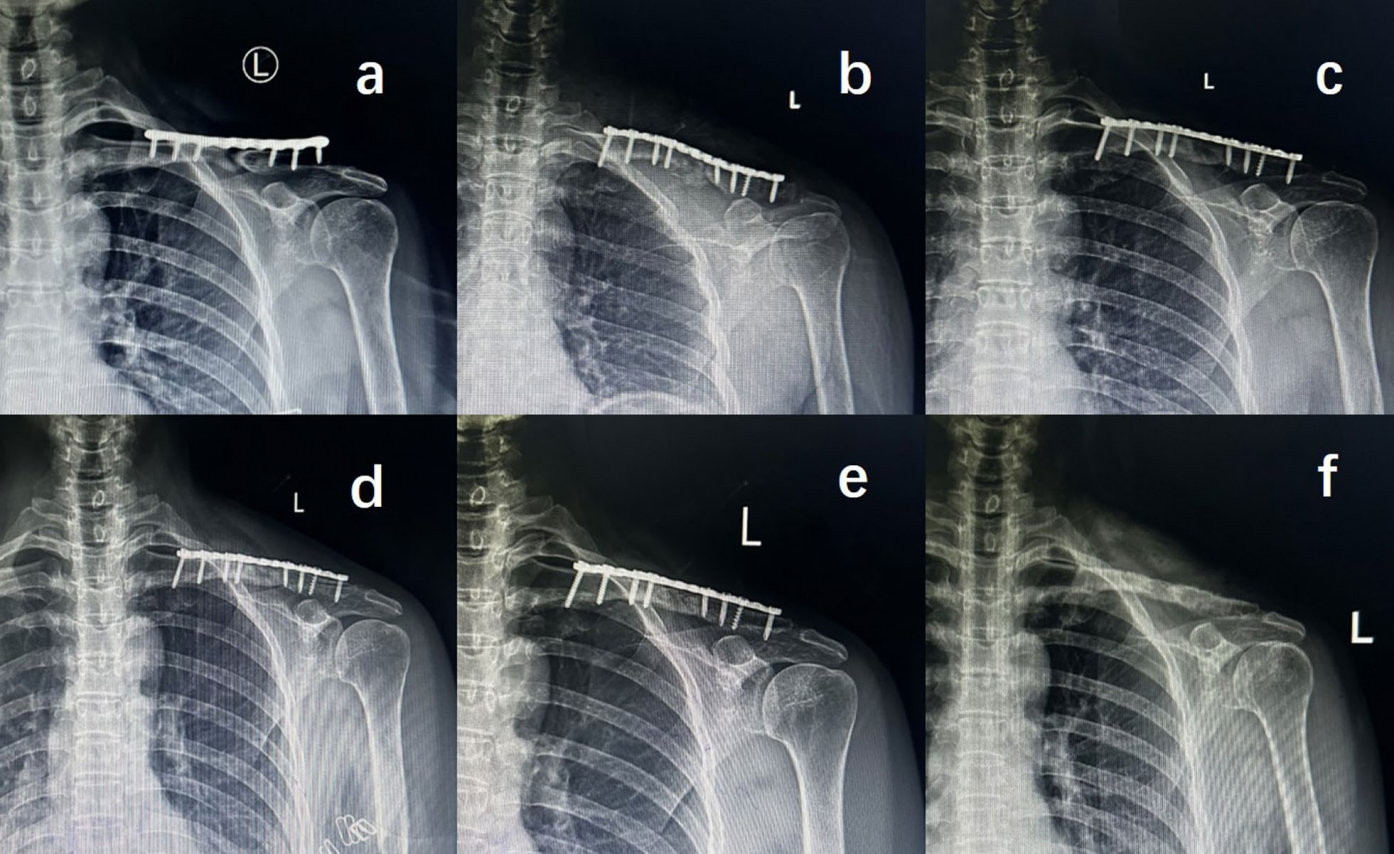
A typical case using the ACGBG technique involving a 54-year-old man with clavicular midshaft nonunion secondary to a fall 2 years prior. a Primitive, b post-revised, c and at 2 months postoperatively. d Plain radiographic images showing the union, and continuous sclerotic bone callus passing through the fracture gap, e at 15 months post-revision surgery showing stable fracture healing, f at day 1 post-plate removal, showing osseous healing at the fracture site. ACGBG, autologous cancellous granular bone grafting
